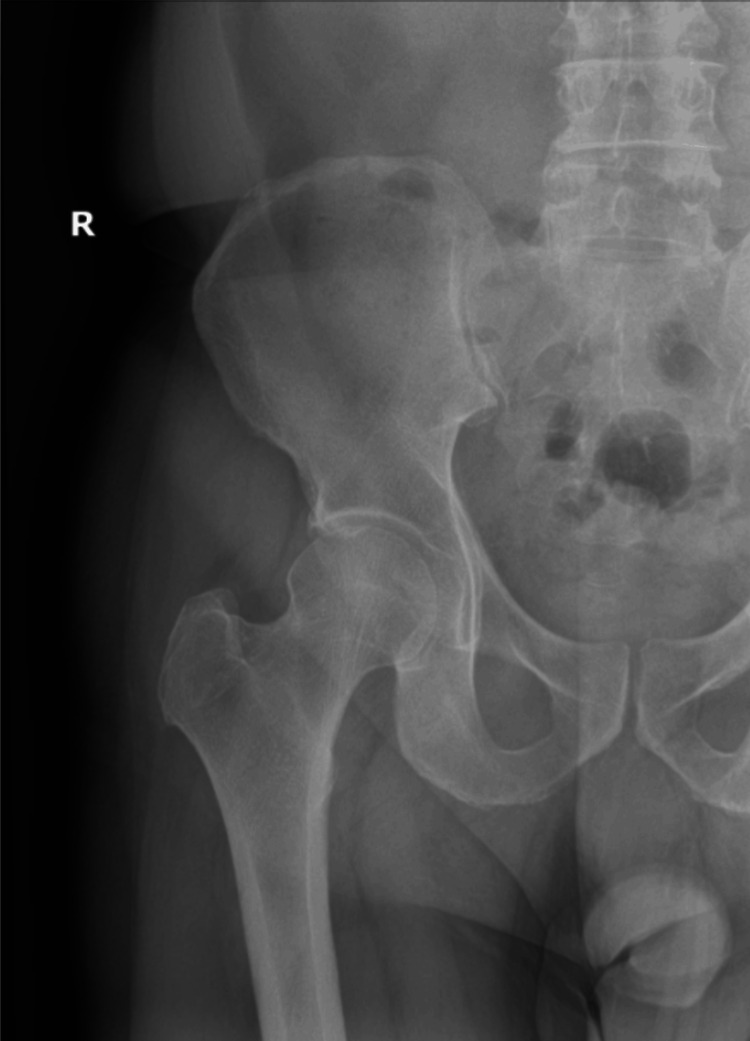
Right hip X-ray. The patient’s right hip X-ray indicates joint space narrowing and osteophyte formation consistent with moderate OA changes.
